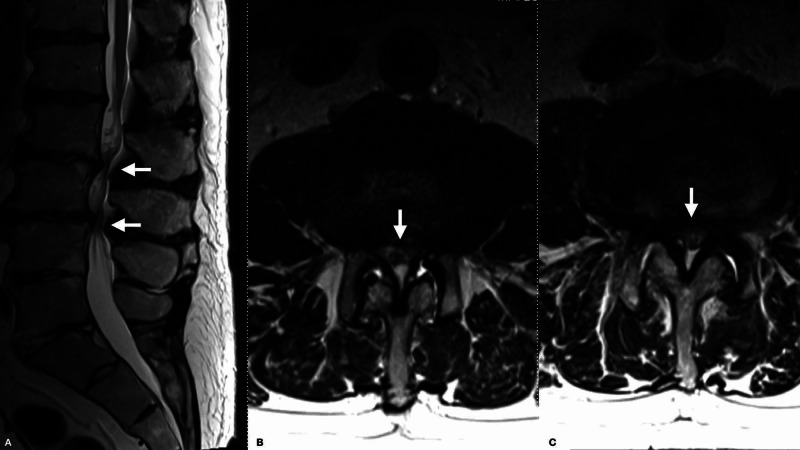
MRI lumbar spine demonstrating spinal stenosis at L2-3 and L3-4 Lumbar spine MRI demonstrating moderate to severe stenosis at L2-3 and L3-4 levels, see arrows identifying areas of severe stenosis. (A) Sagittal T2 image. (B) L2-3 level axial T2 image. (C) L3-4 level axial T2 image.
